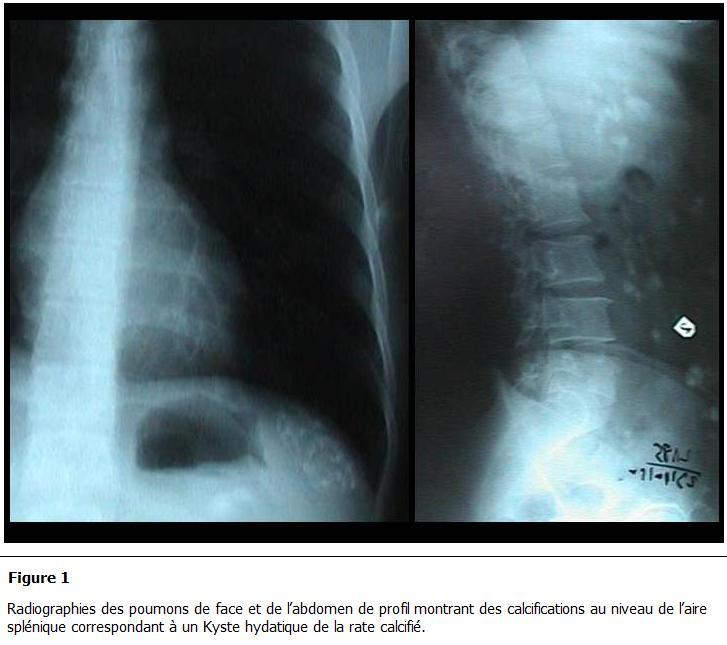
Radiographies des poumons de face et de l’abdomen de profil montrant des calcifications au niveau de l’aire splénique correspondant à un kyste hydatique de la rate calcifié
